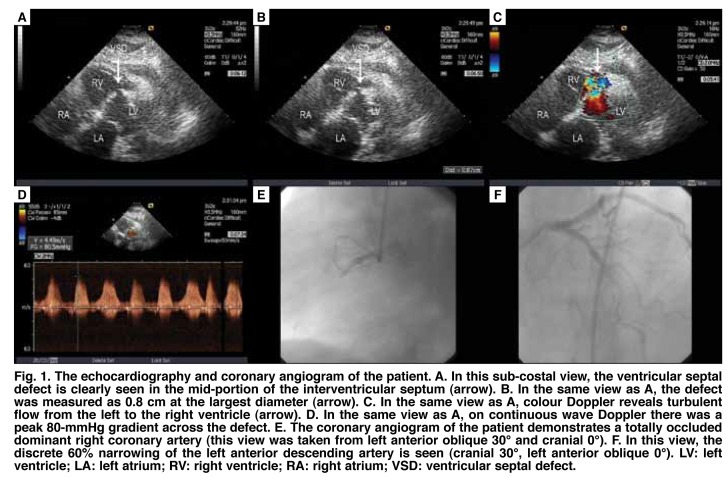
The echocardiography and coronary angiogram of the patient. A. In this sub–costal view, the ventricular septal defect is clearly seen in the mid–portion of the interventricular septum (arrow). B. In the same view as A, the defect was measured as 0.8 cm at the largest diameter (arrow). C. In the same view as A, colour Doppler reveals turbulent flow from the left to the right ventricle (arrow). D. In the same view as A, on continuous wave Doppler there was a peak 80–mmHg gradient across the defect. E. The coronary angiogram of the patient demonstrates a totally occluded dominant right coronary artery (this view was taken from left anterior oblique 30° and cranial 0°). F. In this view, the discrete 60% narrowing of the left anterior descending artery is seen (cranial 30°, left anterior oblique 0°). LV: left ventricle; LA: left atrium; RV: right ventricle; RA: right atrium; VSD: ventricular septal defect.
